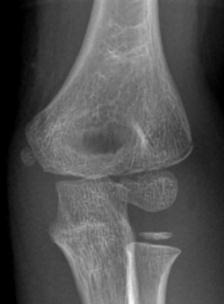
AP radiograph of a Type 1 Jakob Classification lateral condyle fracture.
